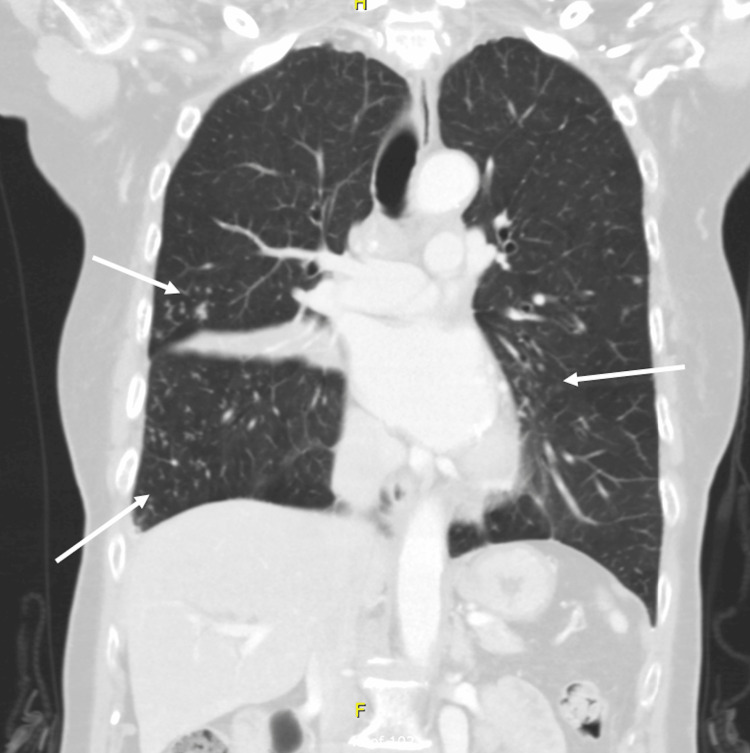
Coronal CT chest showing bilateral, multilobar, tree-in-bud opacities
